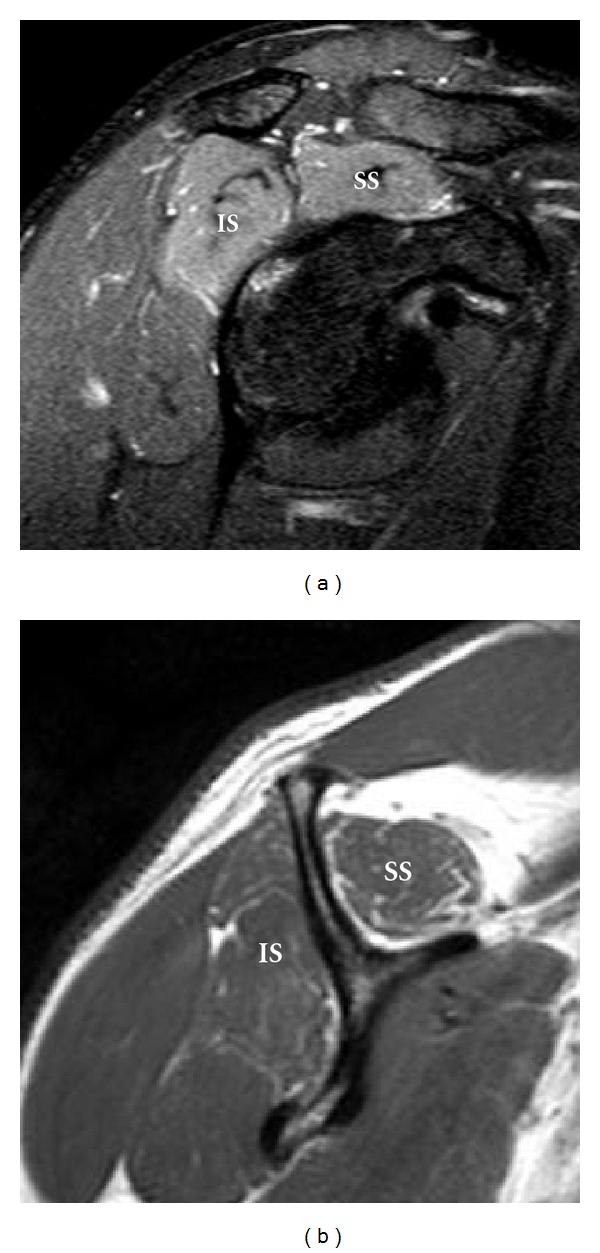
A 44-year-old patient after a swimming accident with clinical and EMG evidence of right supraspinatus and infraspinatus muscle denervation at the suprascapular notch. High signal intensity and mild muscle atrophy with fatty infiltration involving the supraspinatus (SS) and infraspinatus (IS) muscles are demonstrated on sagittal oblique T2 fat-saturated (a) and T1-weighted (b) images.
